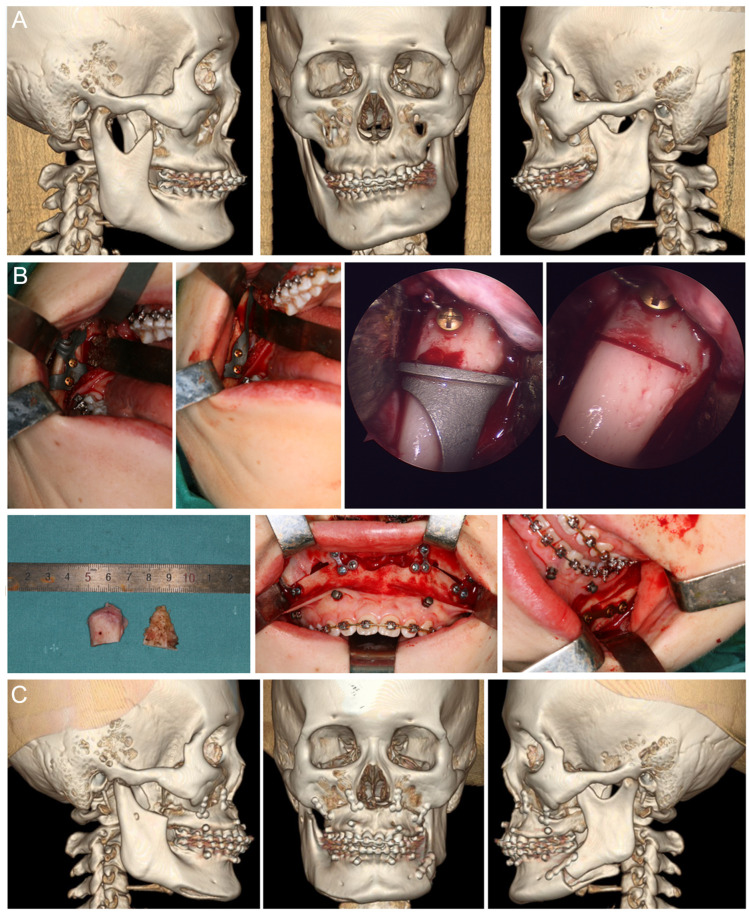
Representative mandibular OC cases treated with the 3D-printed titanium cutting guides: (A) preoperative CT scans showing right mandibular OC, hyperplastic mandibular body and significant chin deviation; (B) intraoperative application of the coronoidectomy cutting guide and condylectomy cutting guide to remove the right mandibular condyle and coronoid according to the surgical plan; moreover, Lefort I osteotomy was performed to correct the secondary maxillary deformity, sagittal split ramus osteotomy was performed on the ipsilesional side in combination with intraoral condylectomy and contour trimming to correct the mandibular asymmetry and malocclusion; (C) reconstruction of the postoperative CT scans showing right mandibular condyle and coronoid were accurately removed according to the surgical plan and patients asymmetry was obviously corrected.
